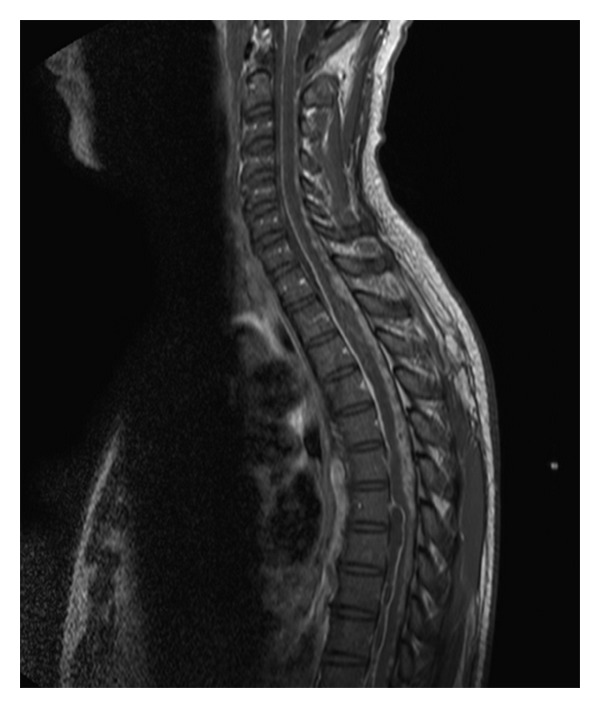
Whole spine T1-weighted MRI with diffuse intradural infiltration of the ependymoma.
